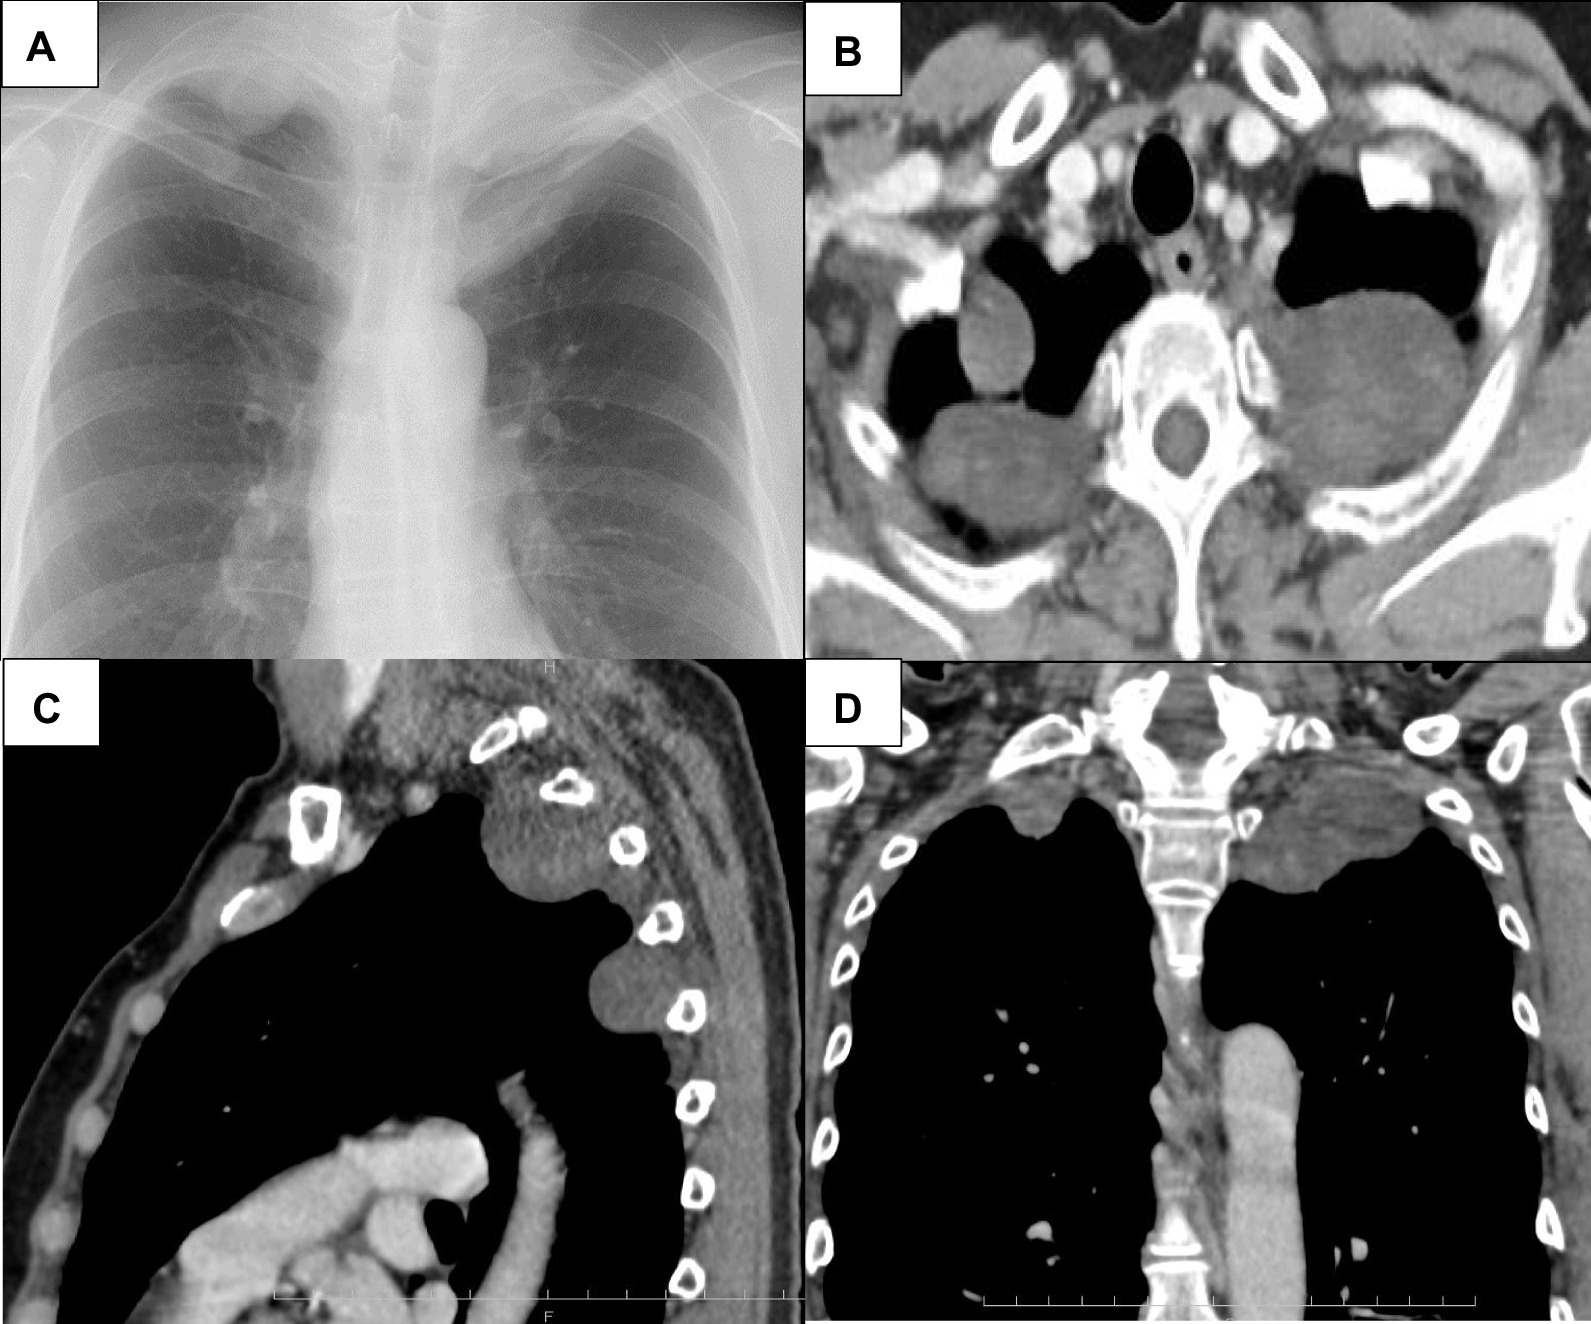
A Chest X-ray shows multiple shadows in both pulmonary apices. B Axial, C sagittal, and D coronal CT view shows well defined, multiple superior mediastinal masses located paravertebrally
